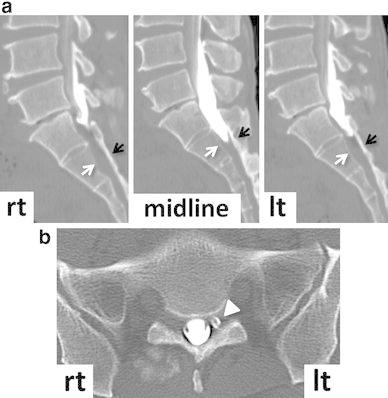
A bone lesion is not seen on sagittal (a) and axial (b) CT myelography. *White* (posterior wall of the vertebral body) and *black* (lamina) *arrows* indicate the bone without lesion (a). *Arrow head* indicates left S2 nerve root (b)
